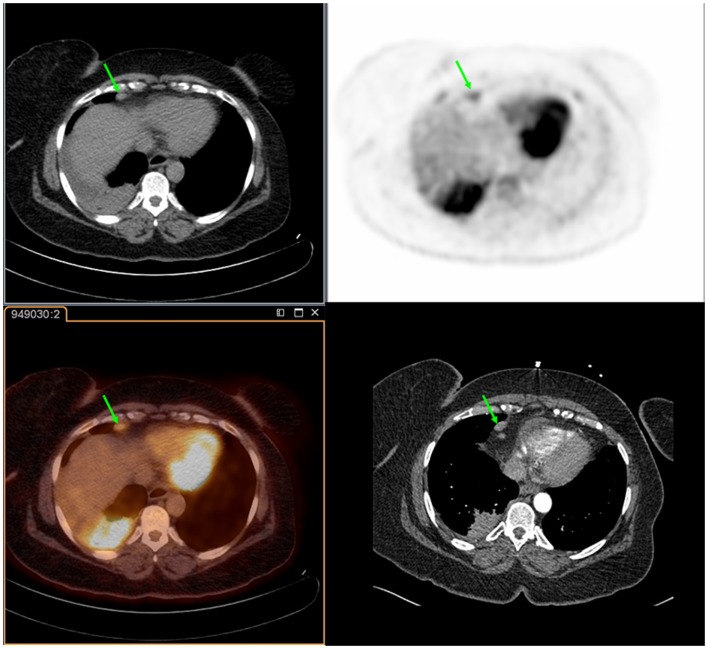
Fifty-six year-old female with a history of squamous cell carcinoma of the right lung. PET/CT demonstrates an FDG-avid cardiophrenic lymph node on non-contrast CT (top left), PET (top right), and fused images (bottom left). These were not commented on in the contrast-enhanced CT (bottom right). Differences in the levels between the two studies are due to patient positioning and respiratory motion.
